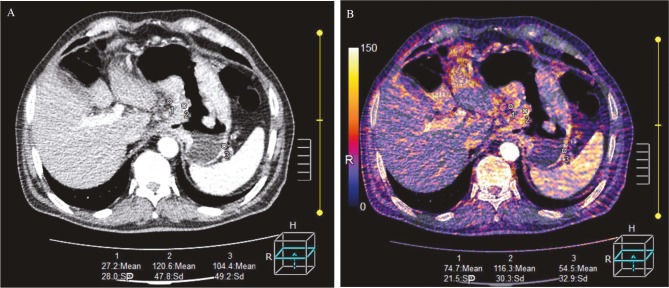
Representative CT images obtained from a 58-year-old female patient with adenocarcinoma with invasion of serosa. (A) The mixed venous phase image shows thickening of the wall of the antrum with transmural enhancement. (B) The iodine shows that the iodine concentrations were 74.7 mg/mL in the perigastric fat (ROI 1), 116.3 mg/mL in the tumor (ROI 2), and 54.5 mg/mL in the normal gastric wall (ROI 3).
